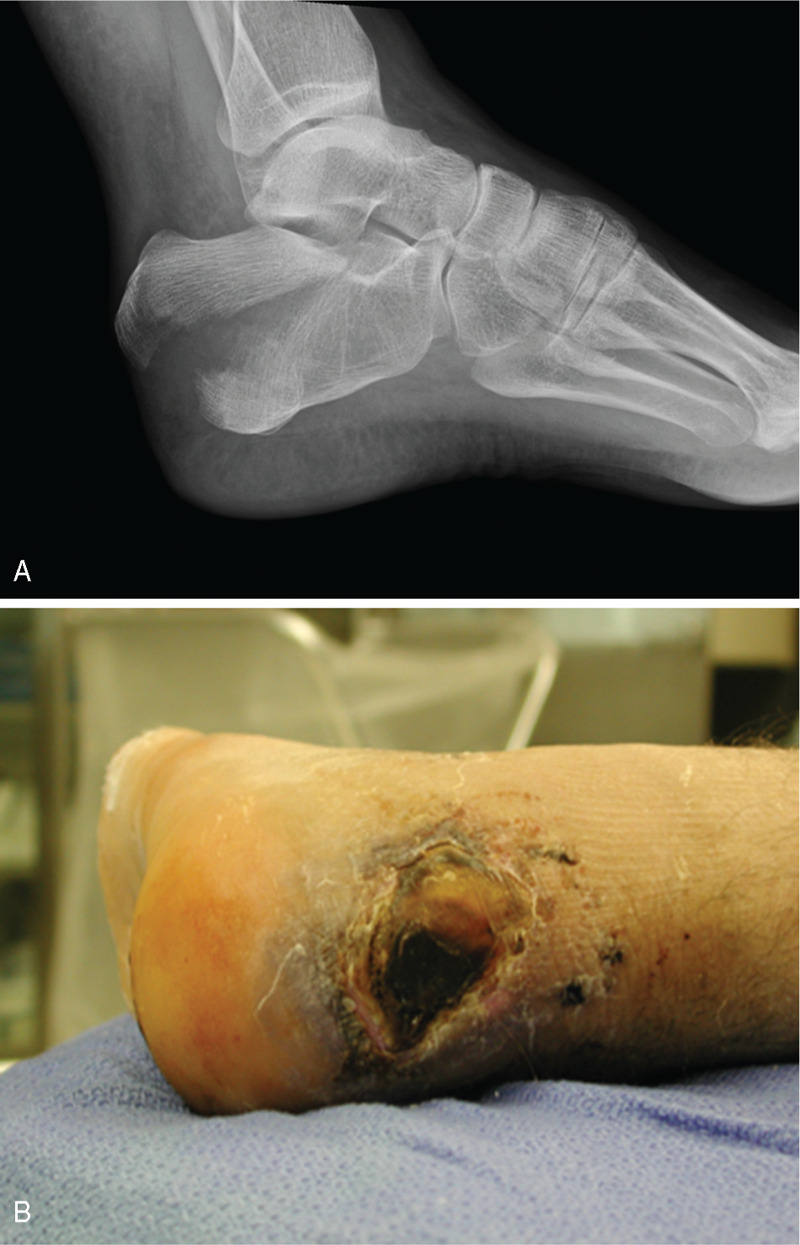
A 22-year-old man fell from a height, sustaining a displaced tongue type fracture (A: lateral foot radiograph). He was initially evaluated at an outside hospital where he was given instructions to return. He presented to our clinic 8 days later with necrosis of the skin surrounding the fracture (B: clinical photograph). Despite urgent reduction and fixation, with local wound debridement and coverage, he developed osteomyelitis.
